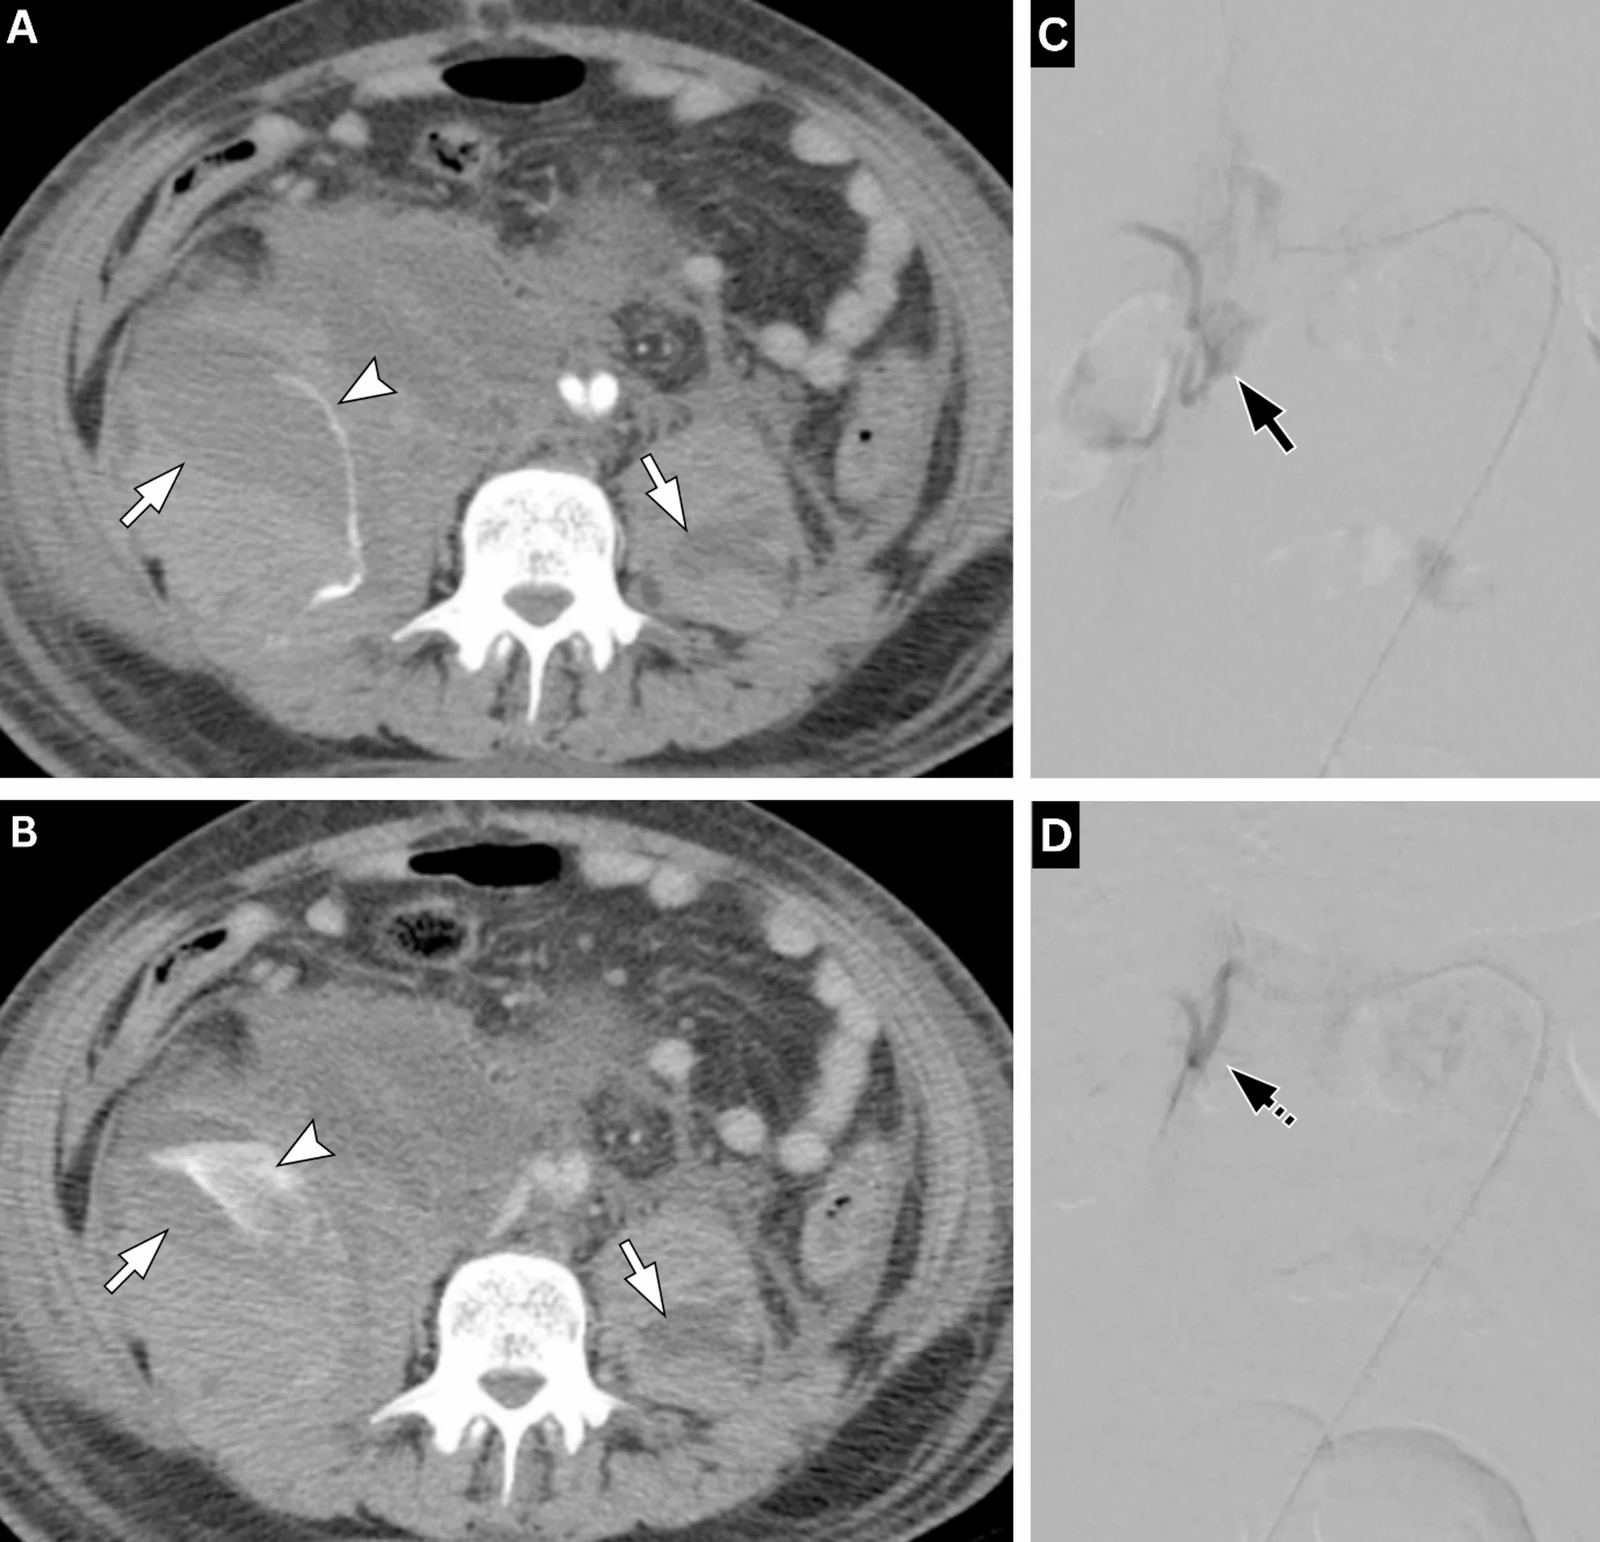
Retroperitoneal hemorrhage due to scrub typhus managed with endovascular therapy in a 52-year-old male who presented with fever, sudden-onset abdominal pain, and hypotension. Axial arterial A and venous B CT (CECT) demonstrates bilateral right greater than left psoas hematomas (solid white arrows) with active contrast extravasation on the right (white arrowheads). C Pre-embolization angiography confirms active contrast extravasation (solid black arrow) from the right iliolumbar artery. D Post-embolization angiogram shows resolution of contrast blush (black dotted arrow)
